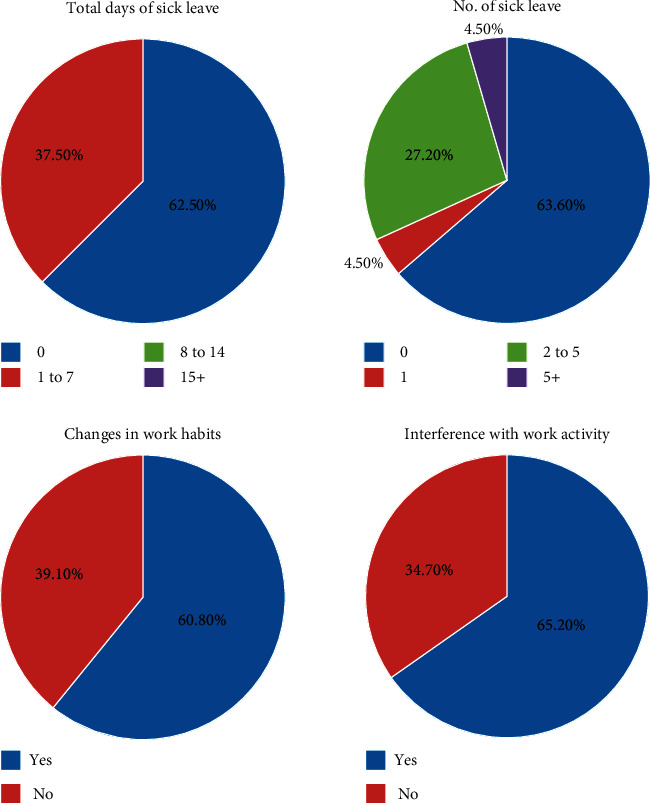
Impact of LBP on radiographers working in Kuwait. Percentage/number values do not always add up to the total percentage/number of questionnaire respondents due to missing data.
